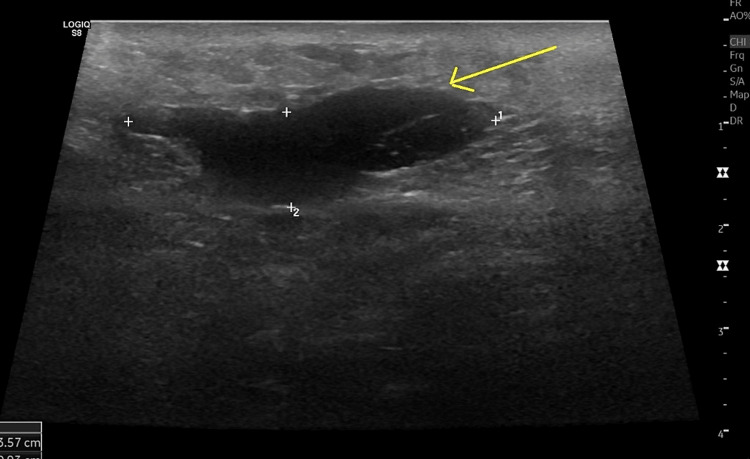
Ultrasound image showing an abdominal wall hematoma (arrow).
